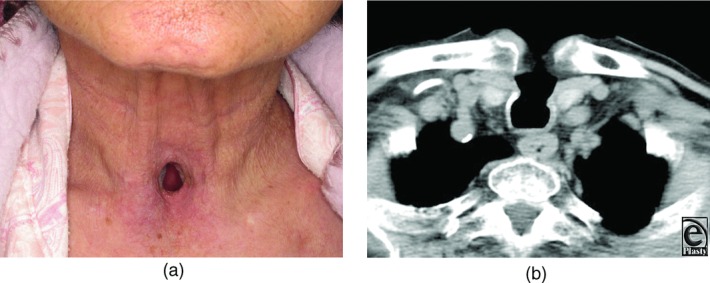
Tracheocutaneous fistula. (a) Preoperative photograph. (b) Computed tomographic scan showing a tracheocutaneous fistula of 15 × 20 mm.
